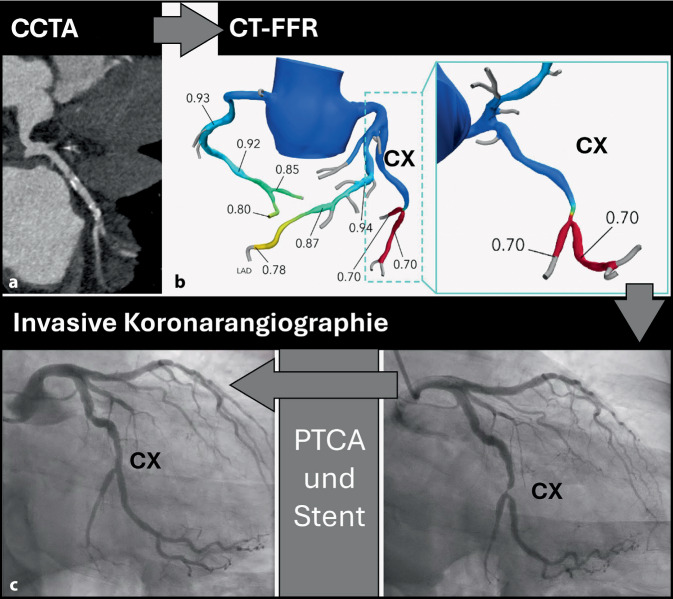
Ein 57-jähriger Mann mit pektanginösen Beschwerden wurde untersucht. a Die Computertomographie-Koronarangiographie (*CCTA*) zeigte eine schwere (70–99 %) Stenose im Ramus circumflexus (*CX*), CAD-RADS 4A. b Die CT-basierte Evaluation der fraktionellen Flussreserve (*CT-FFR*) zeigte Werte ≤ 0,80 und bestätigte somit die hämodynamische Relevanz der Stenose. c In der anschließenden Herzkatheteruntersuchung wurde eine Stenose von über 90 % im Ramus circumflexus/Ramus marginalis 2 festgestellt. Eine perkutane transluminale Koronarangioplastie (*PTCA*) wurde durchgeführt, bei der die Stenose mittels Implantation eines 3 × 18 mm-Stents behandelt wurde
